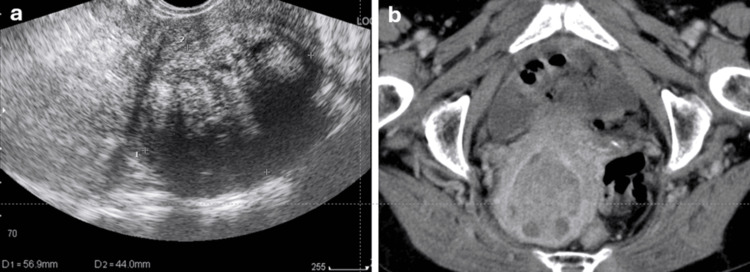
Preoperative imaging findings (a) Transvaginal ultrasonography shows an enlarged uterus with a well-defined echogenic mass occupying the endometrial cavity. The mass demonstrates heterogeneous internal echogenicity, consistent with a solid lesion containing small cystic components. (b) Contrast-enhanced axial computed tomography image demonstrates a well-circumscribed mass filling and expanding the endometrial cavity. The mass shows heterogeneous enhancement relative to the myometrium, with focal non-enhancing areas suggestive of cystic components. No fat density or calcification is observed
